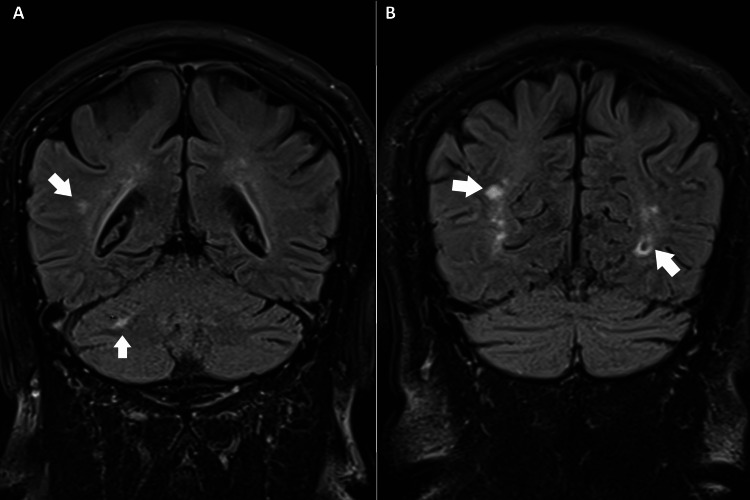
Magnetic resonance imaging (MRI) of the brain A and B) Multiple non-specific, tiny fluid-attenuated inversion recovery (FLAIR) hyperintense foci (white arrows) are observed in the subcortical white matter of the supratentorial brain.
